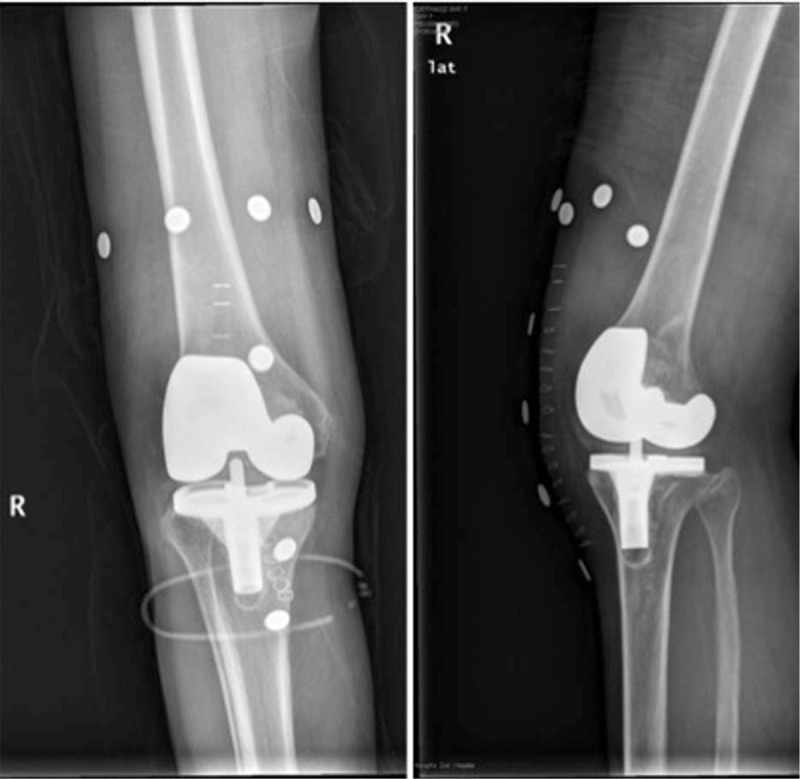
Postoperative check radiographs. Artificial knee prosthesis has been successfully implanted.
